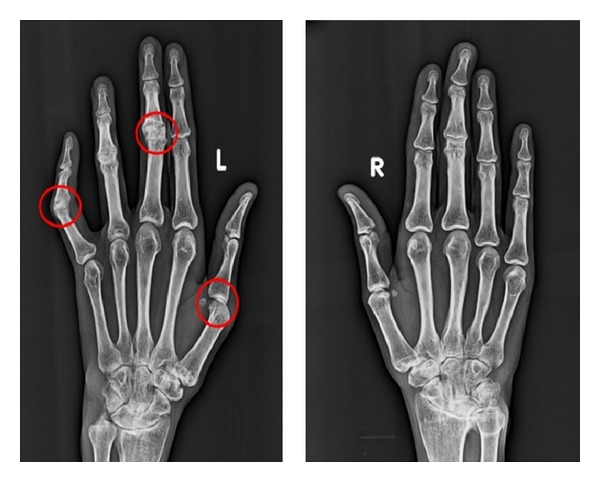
Hand wrist radiograph showing periarticular osteoporosis of interphalangeal joints of fingers.
